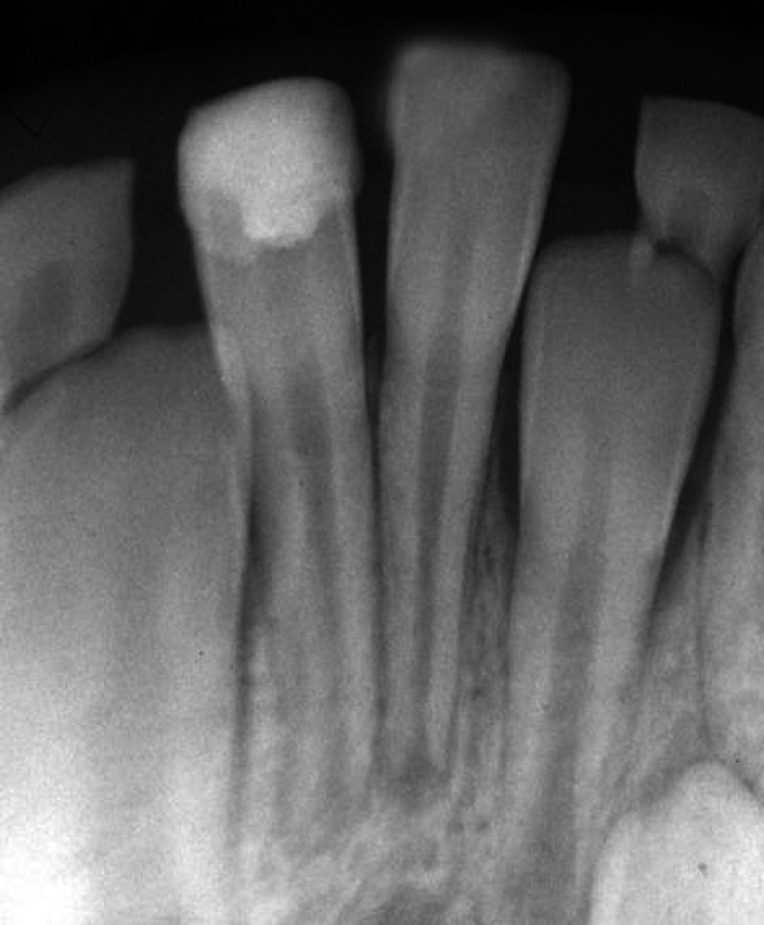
X-ray image of the right central incisor after performing partial pulpotomy
